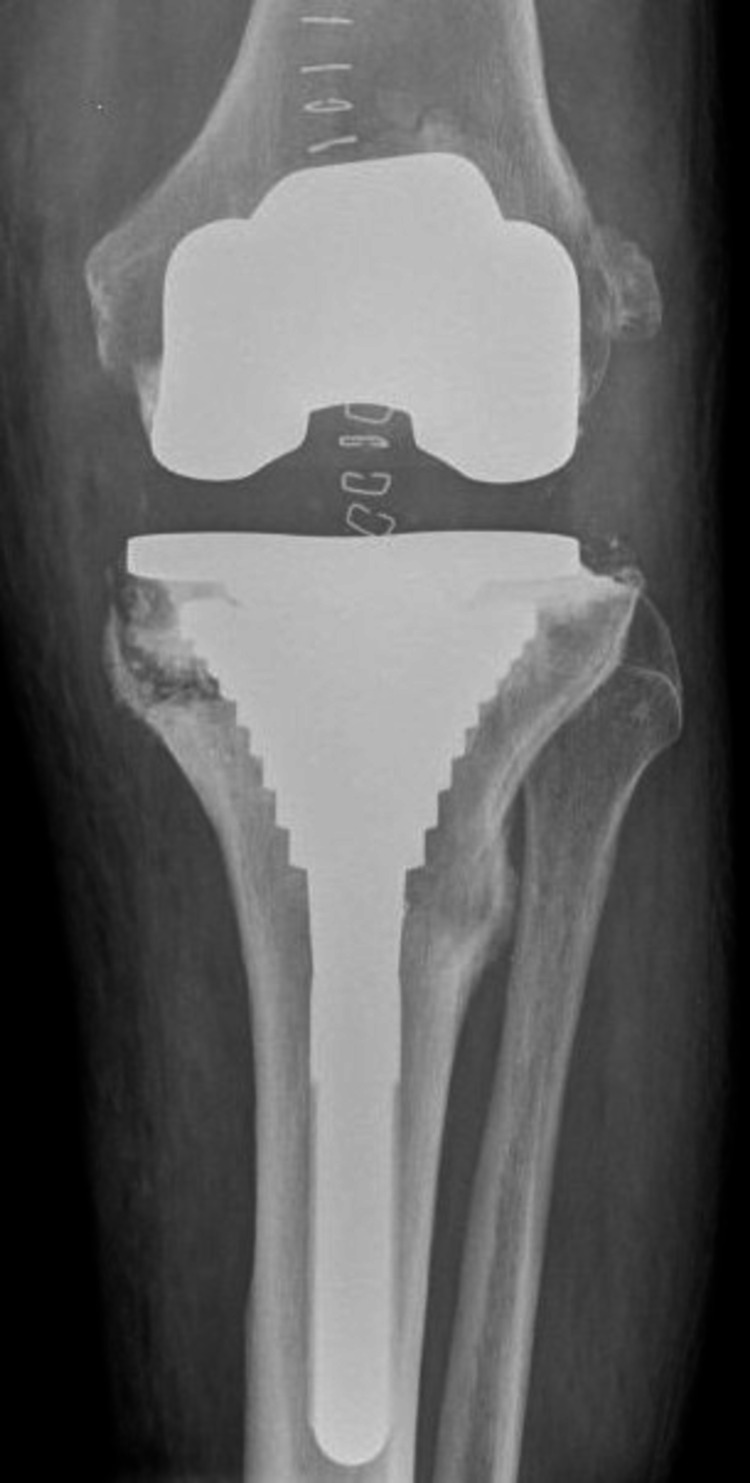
Early postoperative X-ray anteroposterior view showing lack of metal-bone contact in the medial aspect of the metaphyseal sleeve.
